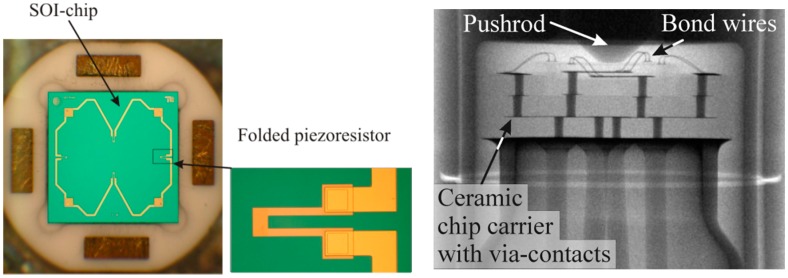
Left—top view of SOI-pressure sensor chip placed in ceramic carrier cavity (dotted line indicates centerboss membrane) with detailed view of folded piezoresistor; Right—X-ray photograph of the packaged sensor. The bond wires, pushrod and the feed through are visible [8].
